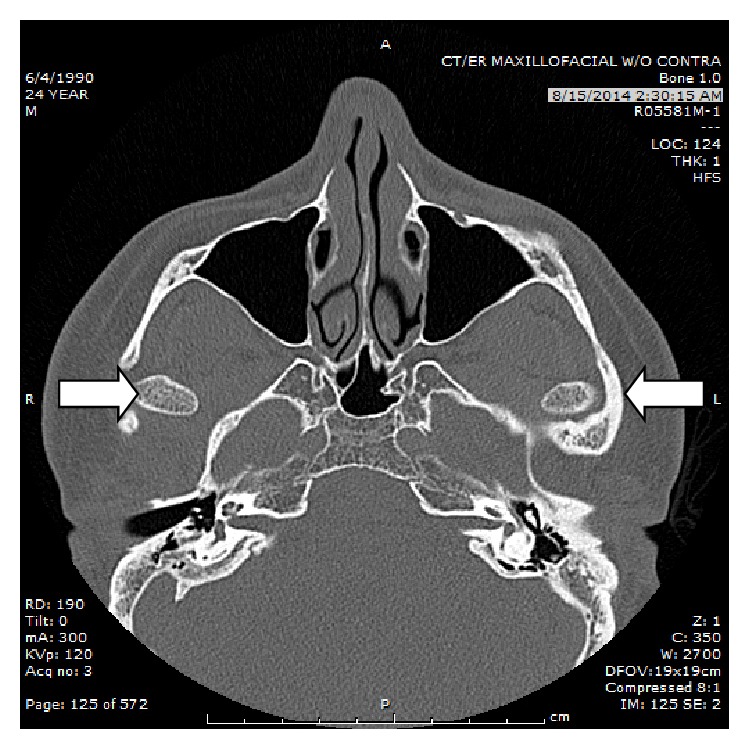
CT showing bilateral condylar heads (arrows) anterior to the glenoid fossa.
